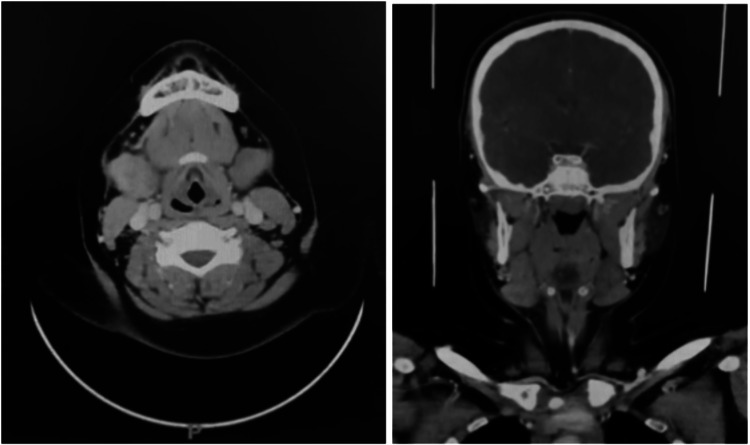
Coronal and axial view of CT scan with contrast show asymmetrical enlargement of the right submandibular gland and no foreign body was identified.
